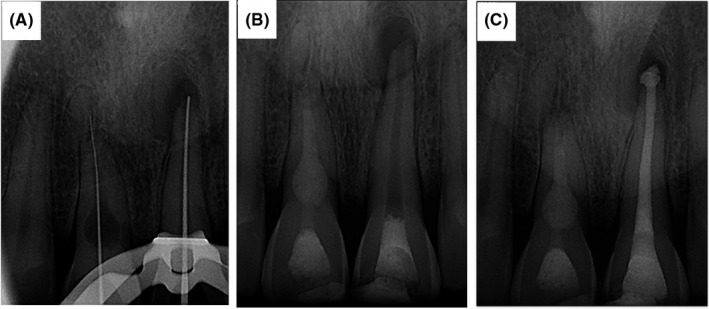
Periapical radiography showing working length determination (A), obturation of tooth #8 with Biodentine (B), and obturation of tooth #9 with MTA (C)
